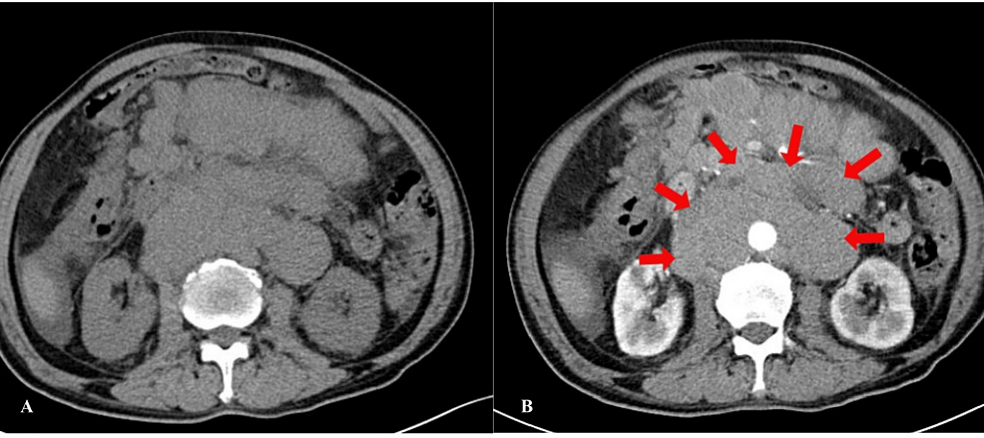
Enhanced CT of the abdomen Enhanced CT of the abdomen and pelvis reveals multiple masses in the abdominal and pelvic fat spaces, retroperitoneum, mesenteric area, and bilateral iliac vessels, possibly indicating manifestations of lymphoma. The abdominal aorta and its branches, portal system, and vena cava system are commonly affected, leading to vascular pushing displacement and compression stenosis.
